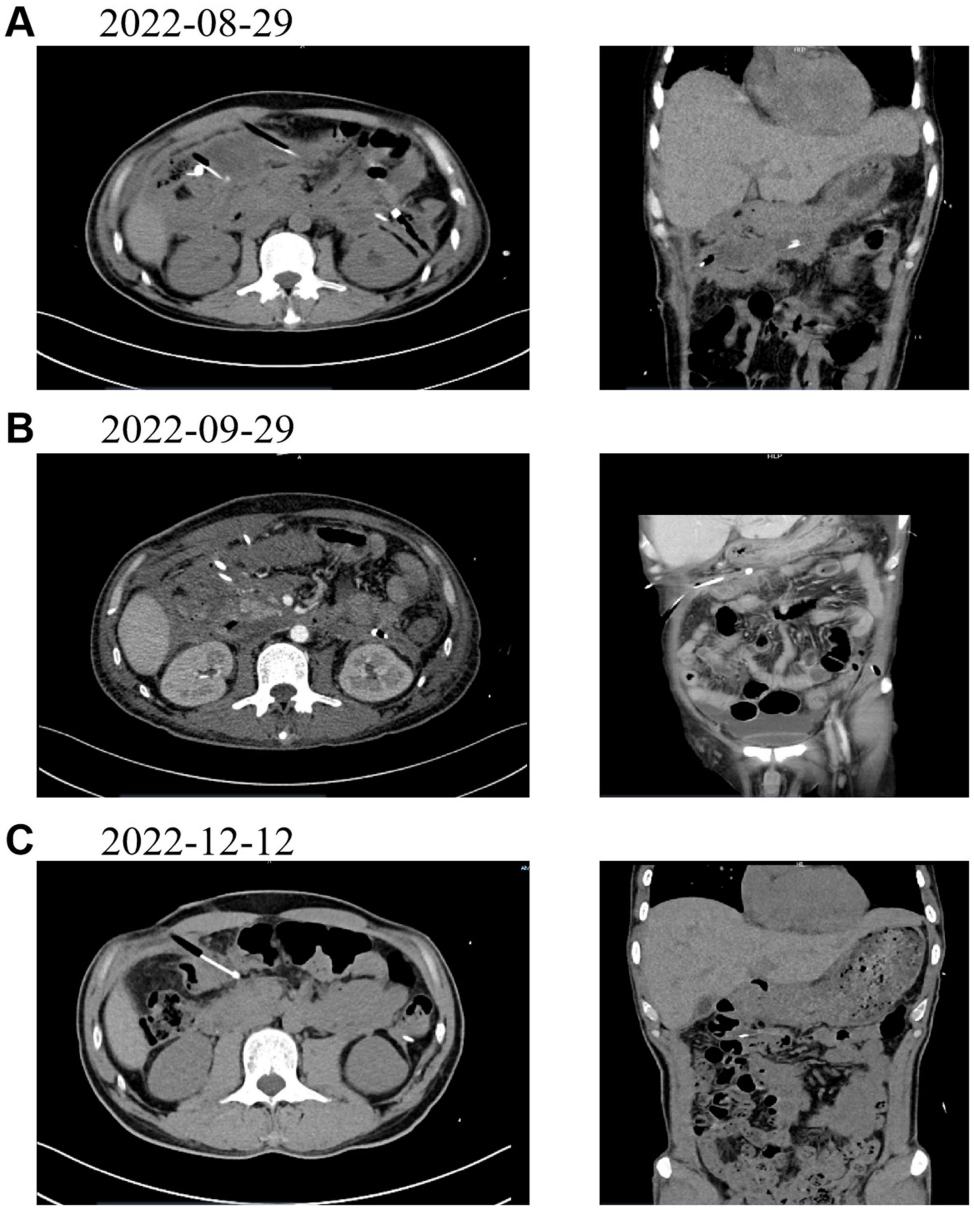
presents follow - up CT images of the patient at various time points post - drainage. (A) (August 29, 2022): CT image taken 1 week after the operation. (B) (September 29, 2022): CT image taken 1 month after the operation. (C) (December 12, 2022): CT image taken 4 months after the operation.
